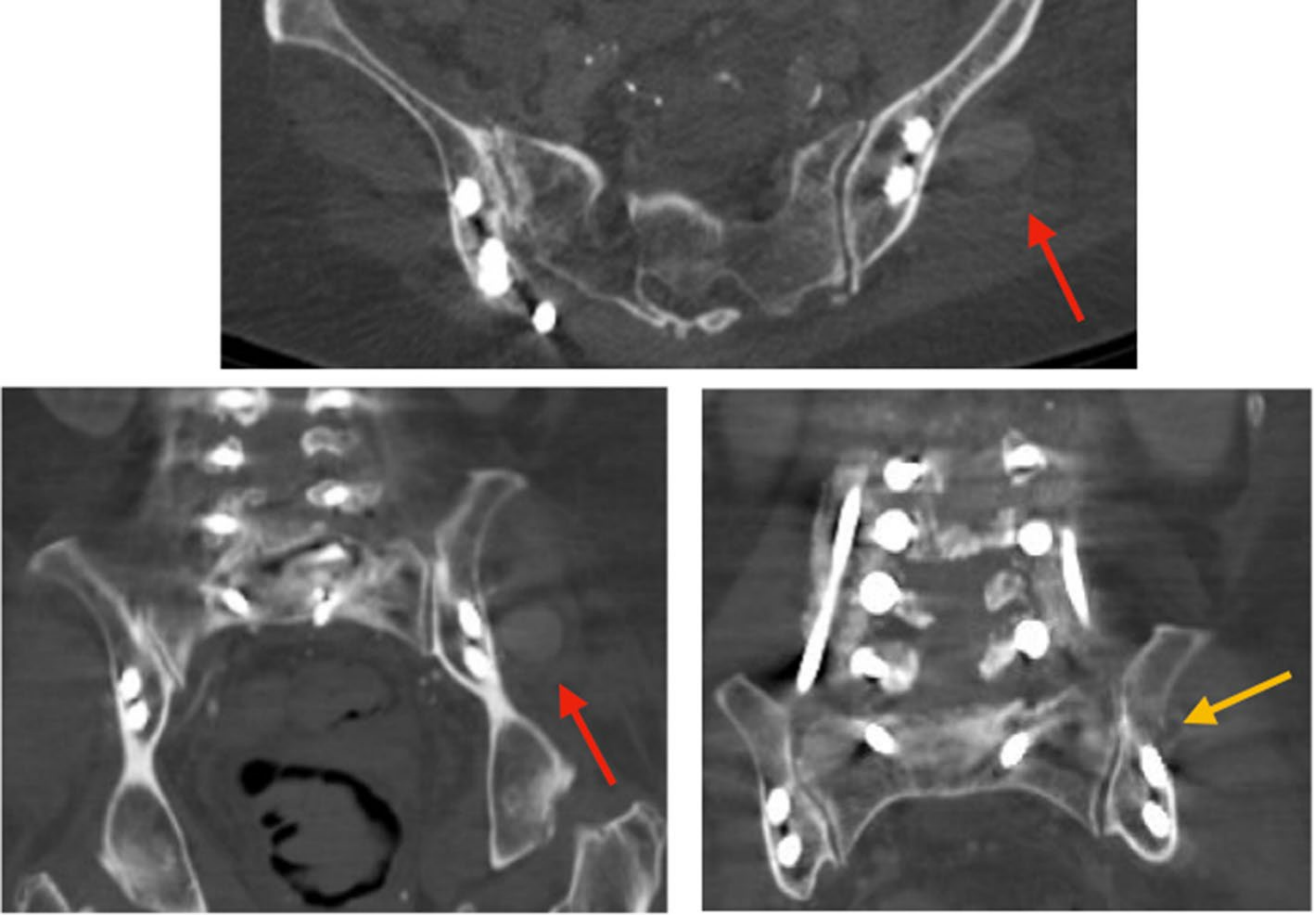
A CT abdomen/pelvis with contrast obtained during re-admission demonstrated an abnormal rounded focus of contrast enhancement adjacent to the left iliac bone measuring up to 2.3 cm × 2.1 cm × 2.3 cm (red arrows). Note the cortical breach of the lateral wall of the ilium (gold arrow) from the original aberrantly planned trajectory for the proximal iliac screw
